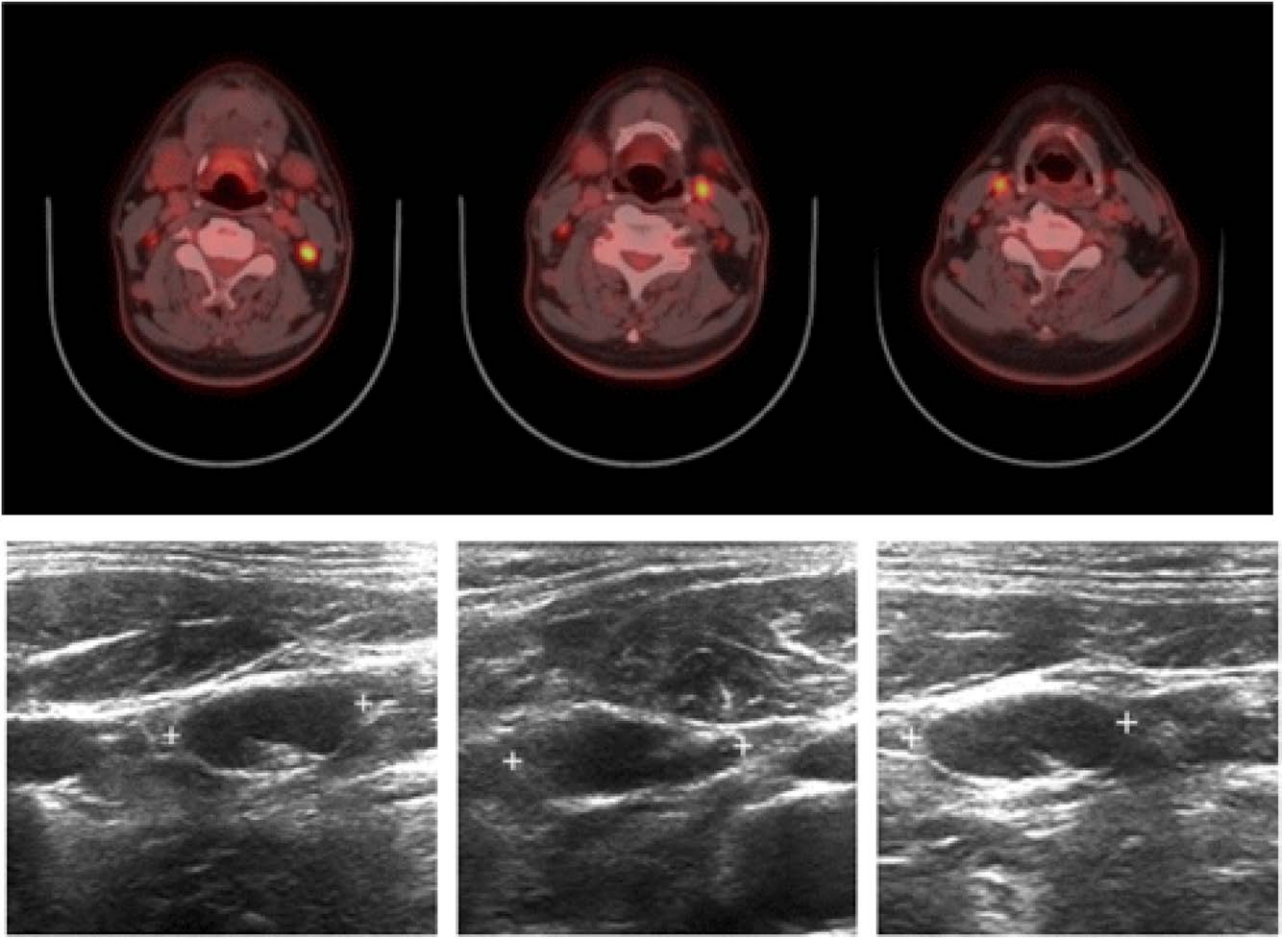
Axial ^18^F-FDG PET and PET/CT images with an abnormal ^18^F-FDG uptake on bilateral neck lymph nodes. Ultrasound shows that the involved nodes are hypoechoic with echogenic thin layers. ^18^F-FDG = ^18^F-fluorodeoxyglucose, PET/CT = positron emission tomography/computed tomography.
